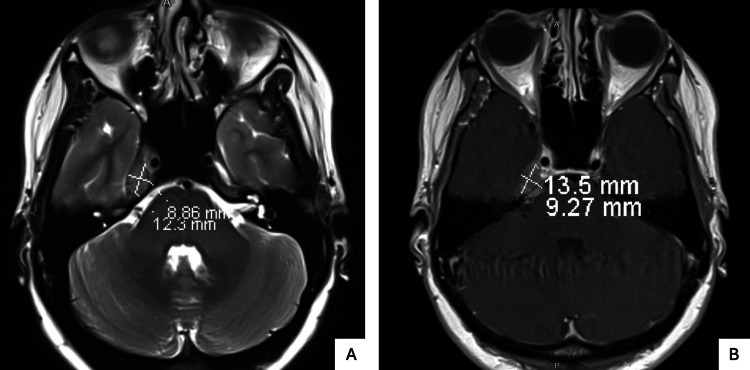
Contrast-Enhanced MRI of the Brain and IACs Revealing a Lesion Encasing the Right Trigeminal Nerve Extending into Meckel’s Cave MRI of the brain and IACs, with and without contrast, demonstrating a well-defined mass measuring approximately 13×9 mm. The lesion partially encases the cisternal segment of the right trigeminal nerve and extends into Meckel’s cave. Panel A shows the mass on axial T2-weighted imaging; Panel B shows post-contrast enhancement on axial T1-weighted imaging. IACs: Internal auditory canals.
